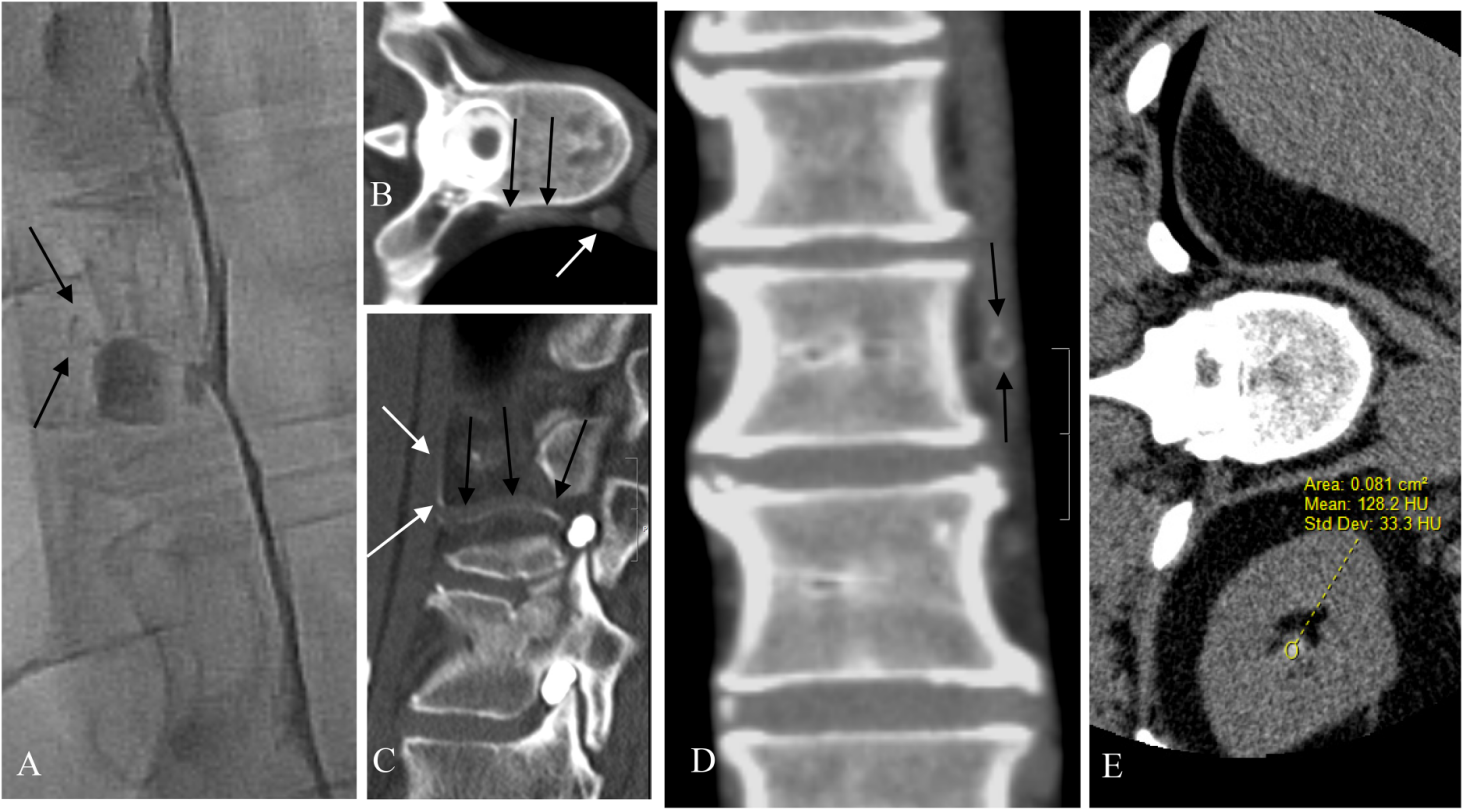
Patient 9. Showing direct CT evidence of CVF. (A) Image from left LDDSM demonstrating subtle evidence of a CVF with faint opacification of a paravertebral vein (black arrows) found retrospectively after seeing the fistula on subsequent LDCT. (B and C) Axial and sagittal reformat of the left LDCT performed immediately following the left LDDSM. Partial opacification of the paravertebral vein (black arrows) can be seen extending from the site of the fistula beside the diverticulum to the hemiazygos vein (white arrow). (D) Coronal reformat of the left LDCT showing the partial opacification of the paravertebral vein. Note the laminar flow effect with persistence of the contrast at the periphery of the vein (black arrows). (E) The left LDCT reveals indirect evidence of the presence of a CVF with early contrast agent arrival in the renal pelvis measuring 128 HU.
